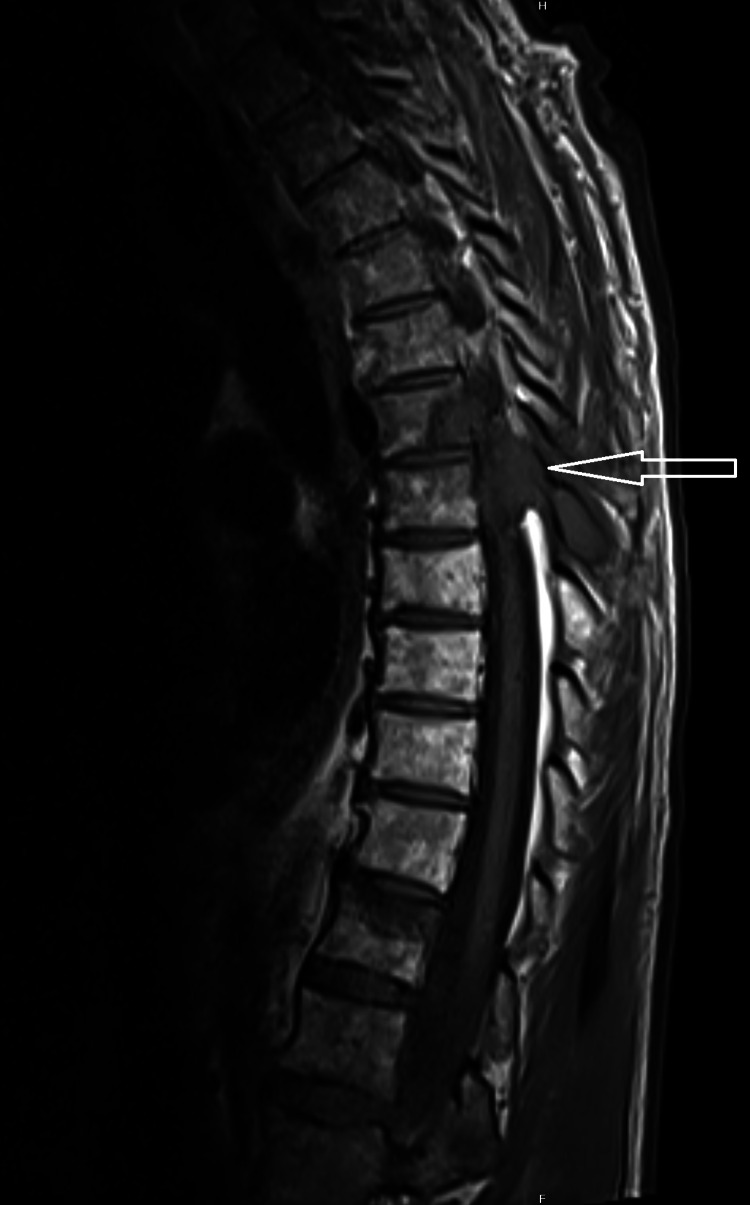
Magnetic resonance imaging of the thoracic spine at the time of lung cancer progression Sagittal view demonstrates a mass at T6-7 extending from the medial left rib into the T6 and T7 vertebral body and extending into the spinal canal with contact and displacement of the spinal cord measuring approximately 7 cm × 4.4 cm greatest dimensions in the axial plane and greatest vertical dimension approximately 4.7 cm (white arrow). Anteriorly this lesion abuts the posterior wall of the thoracic aorta.
